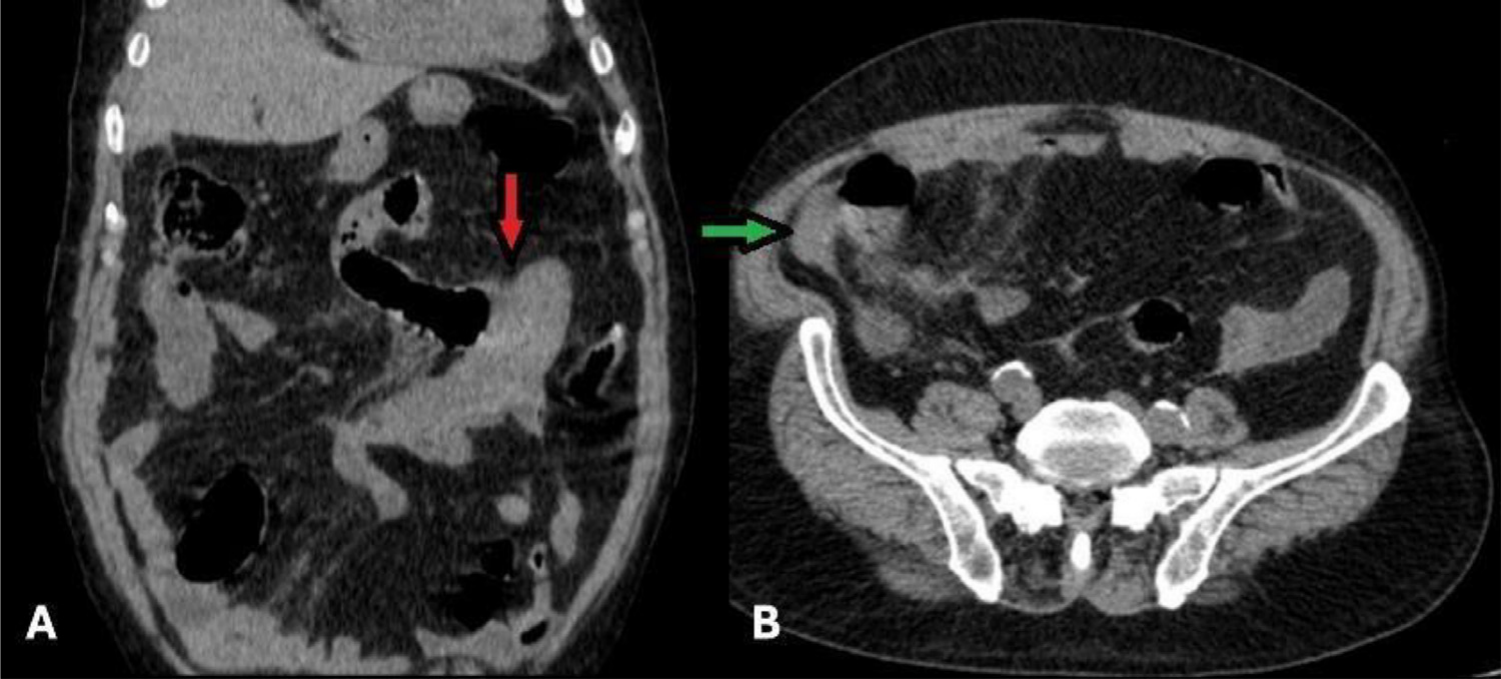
Nonenhanced Abdominopelvic computed tomography (CT) images. (A) Coronal view demonstrates a jejunal loop with circumferential long-segment wall thickening, appearing hyperdense, consistent with an intramural hematoma (IMH). (B) Hyper-attenuated free fluid in the right para-colic gutter, indicative of mild hemoperitoneum, is observed (green arrow).
